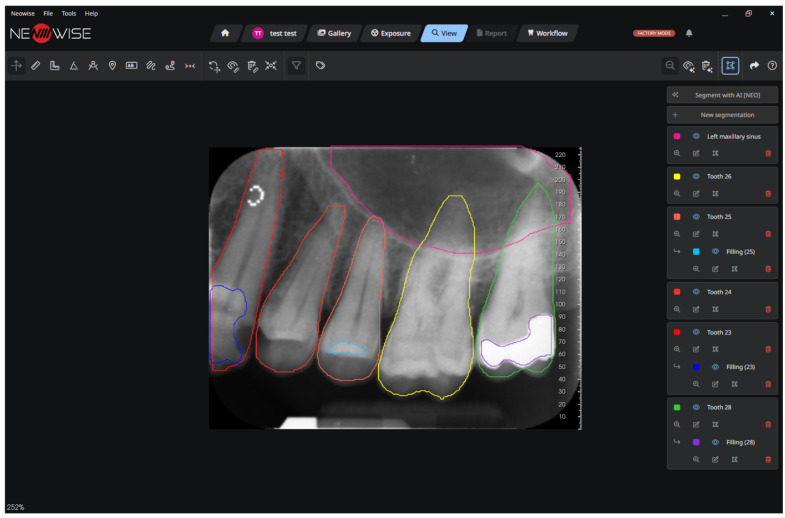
Periapical segmentation.
